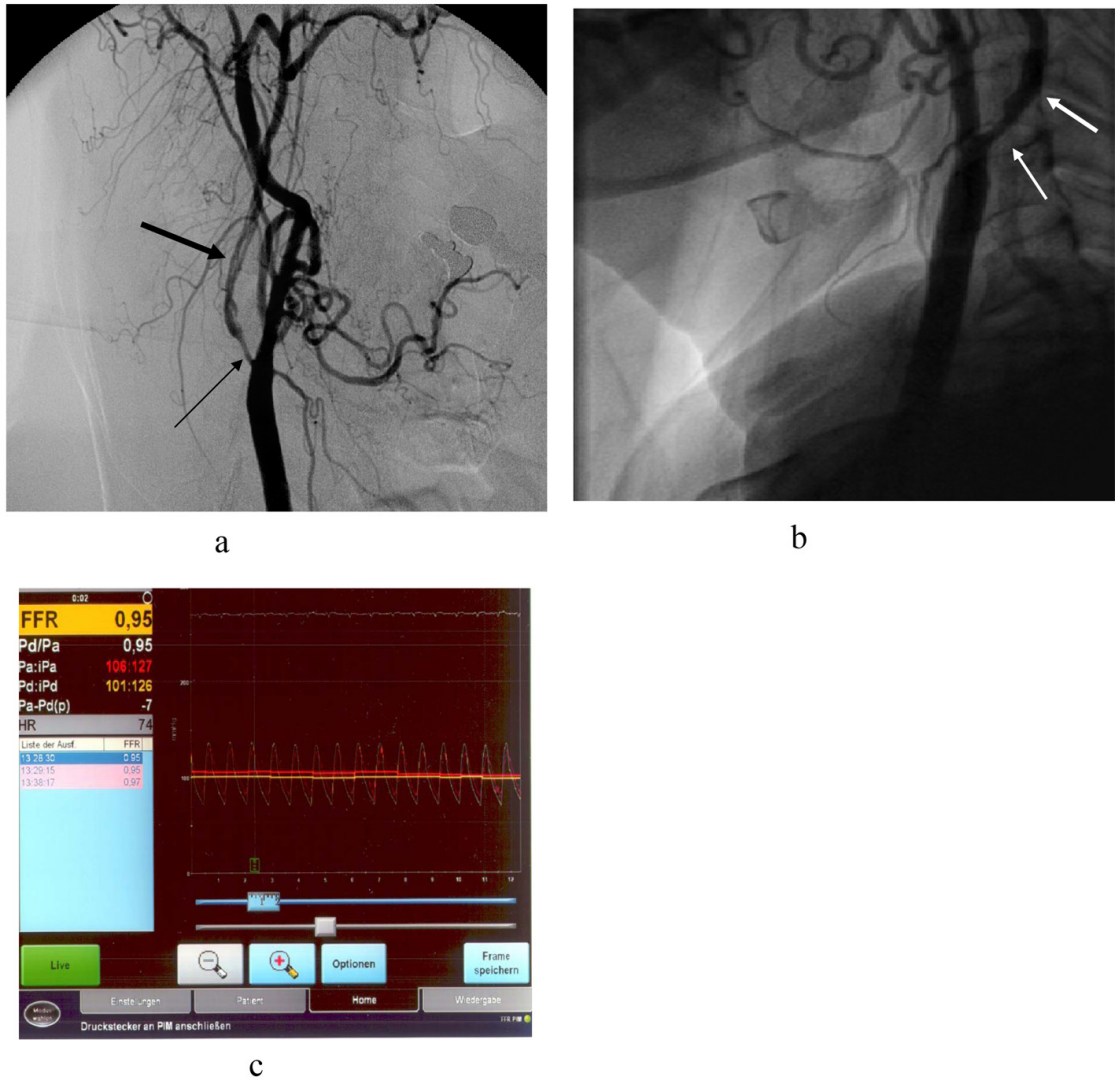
(a). Symptomatic subacute dissection (thick arrow) of the right internal carotid (thin arrow) in a 64-year-old woman; (b). Repeat angiography one and a half year later, indicated after sonographic diagnosis of severe restenosis, shows only moderate intimal hyperplasia (arrow) and restenosis; (c). Pressure gradient (0.95) measurement across the lesion confirms good functional result. The patient is discharged without undergoing the planned PTA and drug eluting balloon treatment.
